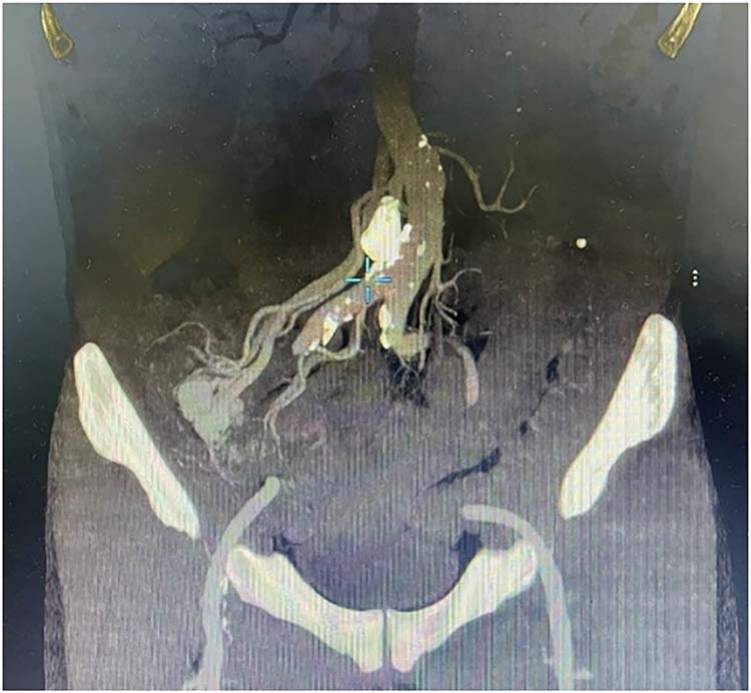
Contrast-enhanced abdominal CT showed a thickened appendix without signs of inflammation and images of vascular proliferation and dilated veins around the appendix.
